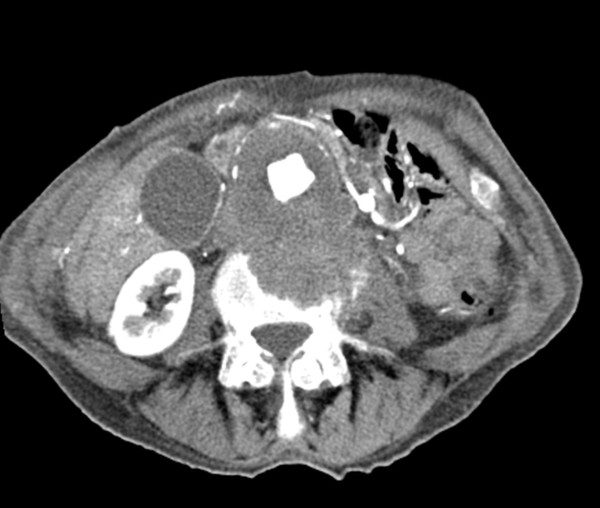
Arterial phase computed tomography (CT) image of the erosion of the fourth lumbar vertebra.
